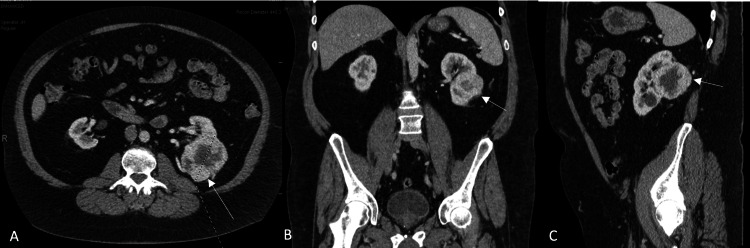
Left renal mass on contrast CT. The solid white arrows indicate the left renal mass in the transverse (1A), coronal (1B), and sagittal plane (1C).
